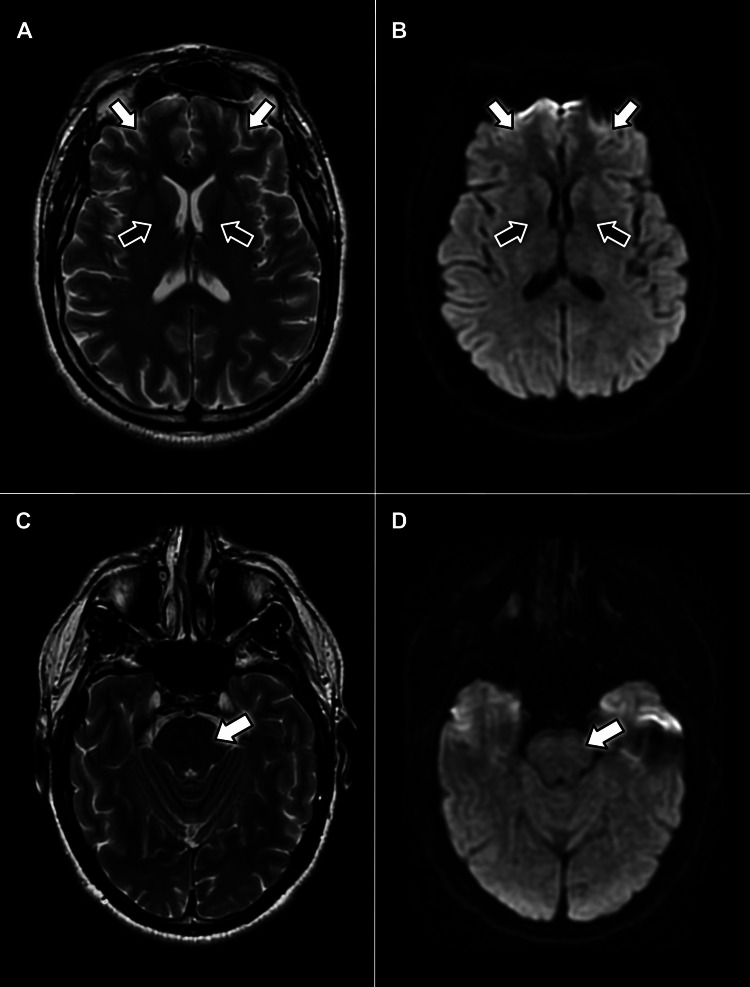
Axial MRI scans without contrast displaying no evidence of pathology in the facial motor cortex (white arrows) or neuron pathways in the internal capsule (black arrows) on T2-weighted (A) or diffusion-weighted (B) sequences. Additionally, no hyperintense signals appear within the pons on T2-weighted (C) or diffusion-weighted (D) sequences
